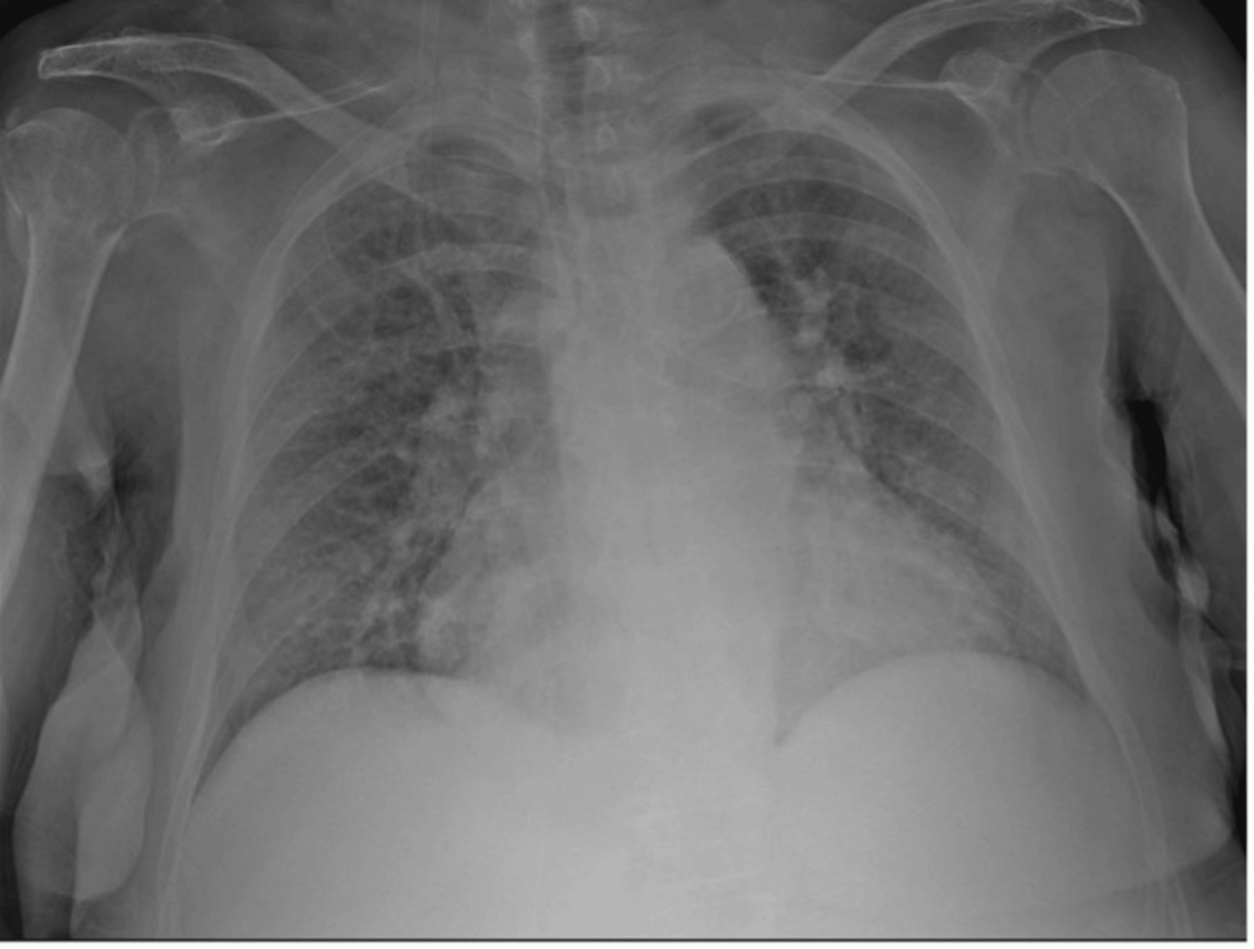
Chest X-ray with bilateral opacities
